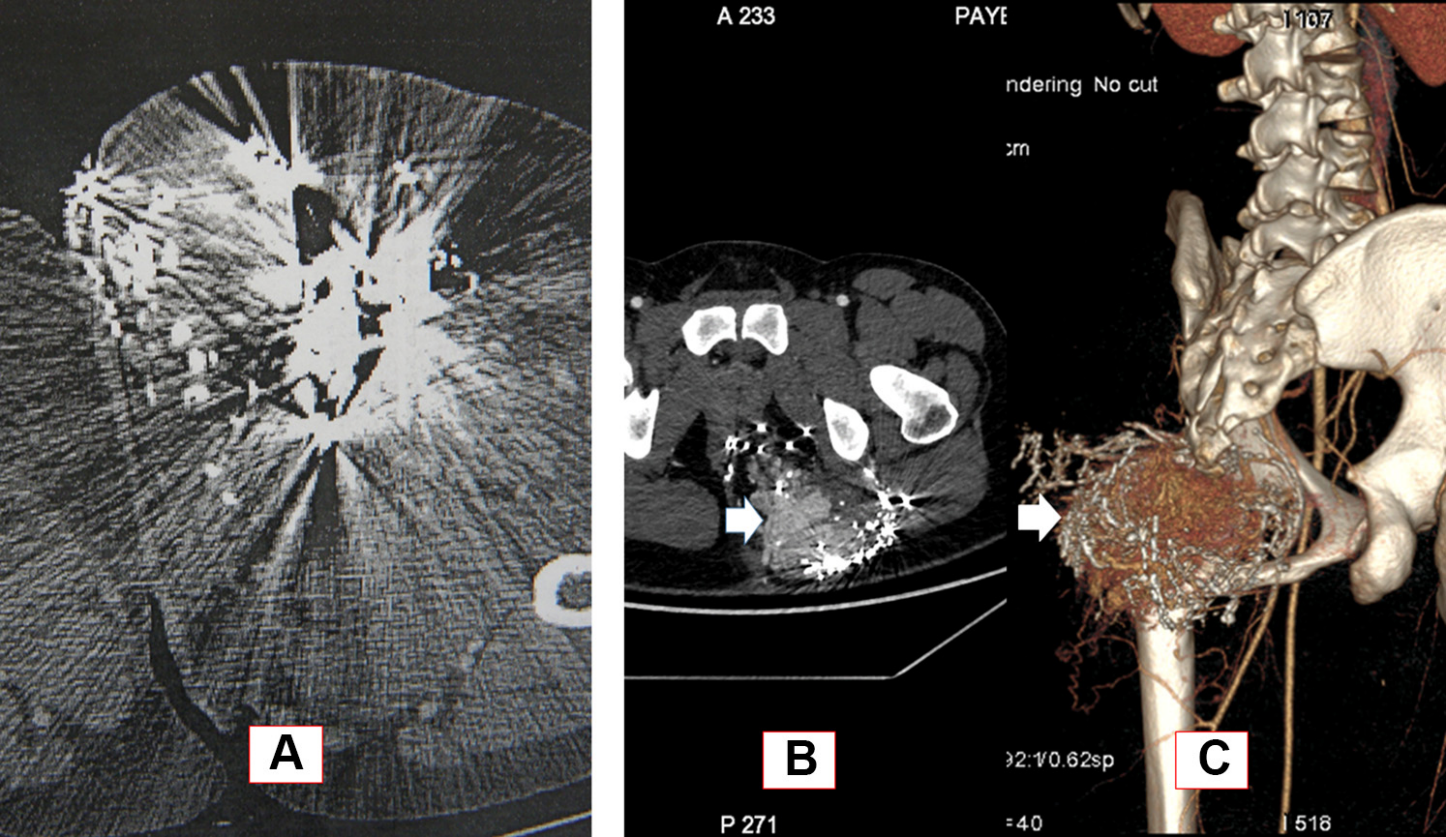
Disadvantadges of embolization with Onyx agent. A, MRI after embolization with Onyx and diffuse artefacts. B and C, Residual arteriovenous malformation (AVM) after Onyx embolization, with a ring of Onyx around the residual nidus in the ischio-rectal fossa.
